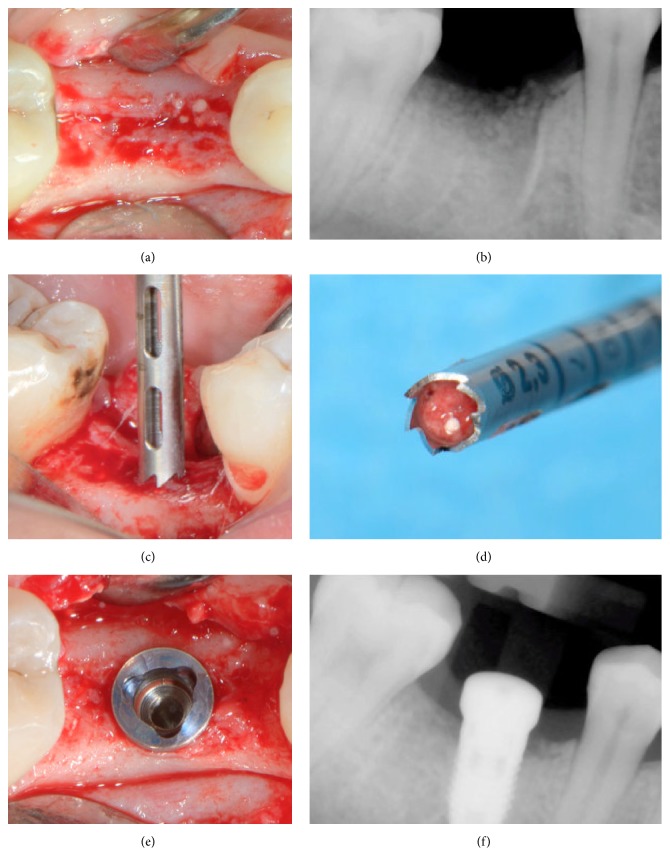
Surgical reentry at 4 months. Intraoperative picture (a) and periapical X-ray (b) showing new bone formation; trephine biopsy (c, d); implant placement at the optimal positioning (e, f).
